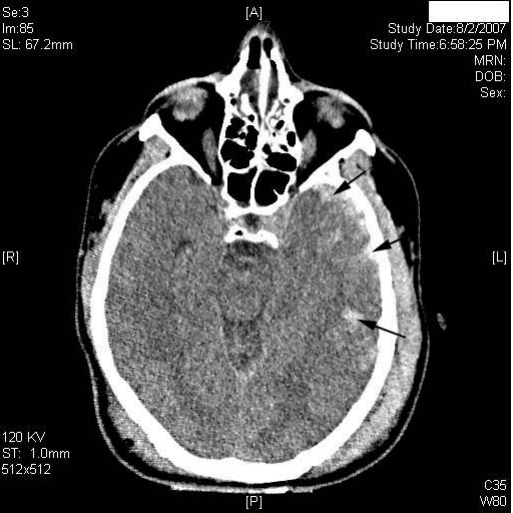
Computed tomography scan at the level of the midbrain. Multiple contusions involving the left temporal lobe are evident (arrows). A = anterior; P = posterior; L = left; R = right.
